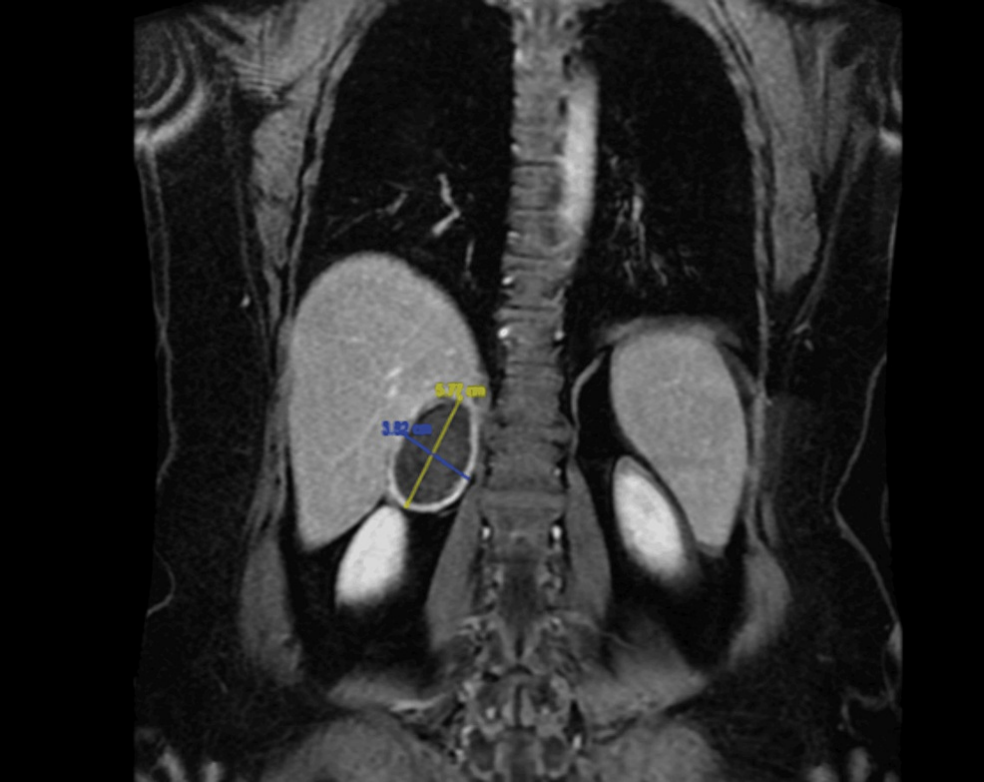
Coronal section of MRI abdomen showing the maximum dimension of the right adrenal mass (T2-weighted)
